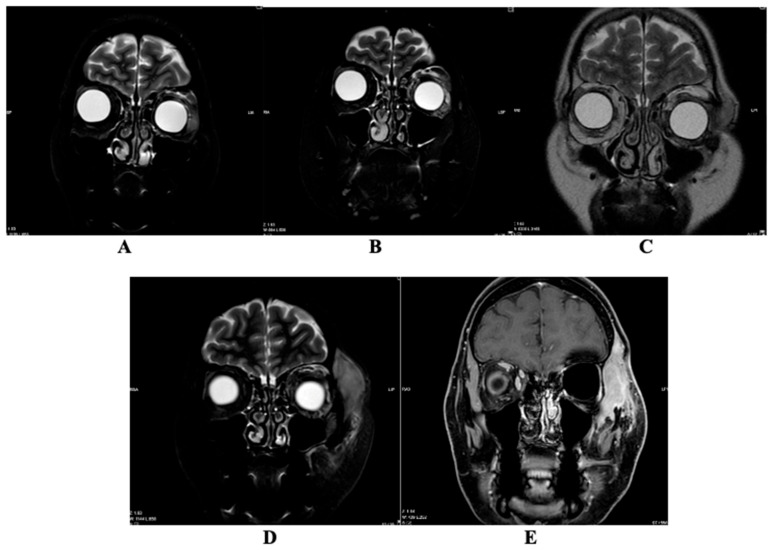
Magnetic resonance imaging (MRI) changes on a coronal plane showing lesion-related changes in the left orbital region. (A) MRI performed 21 December 2022; (B) MRI performed 7 June 2023; (C) MRI performed 27 May 2024; (D) MRI performed 14 August 2024; (E) MRI performed 8 January 2025.
